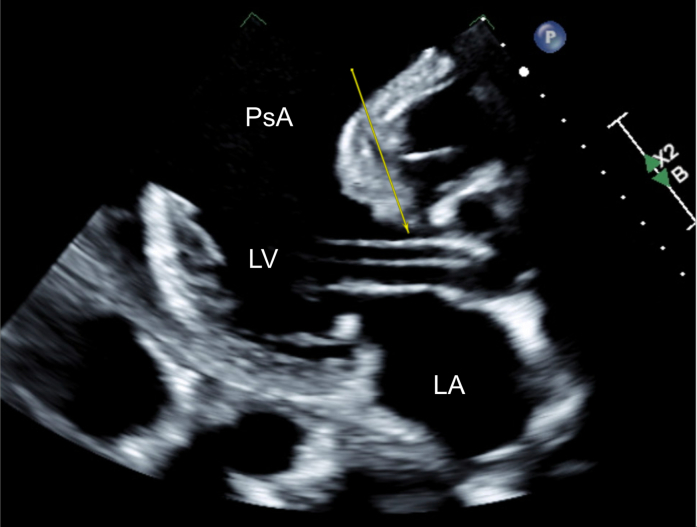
Transthoracic Echocardiography Demonstrating Impella Positioning Parasternal long-axis transthoracic echocardiographic view demonstrating the Impella device (yellow arrow) positioned within the left ventricle. A large left ventricular pseudoaneurysm is partially visualized. LA = left atrium; LV = left ventricle; PsA = pseudoaneurysm.
